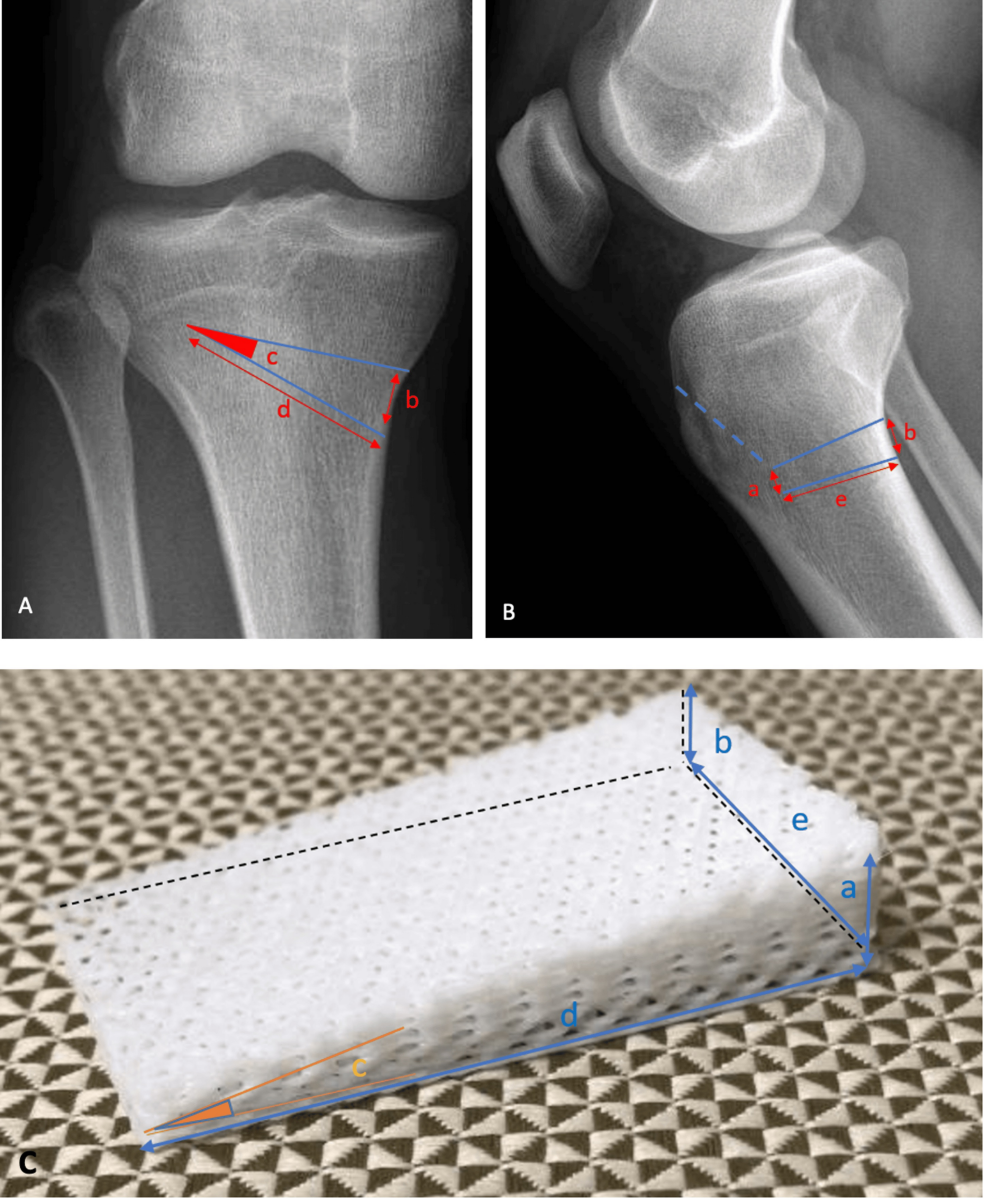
Required dimensions for the bone spacer wedge. A: anteroposterior knee radiograph with the bone wedge dimensions overlayed; B: lateral knee radiograph with the bone wedge dimensions overlayed; C: image of the Osteopore bone wedge used in high tibial osteotomies with labelled dimensions; a: anterior wedge height (conventionally measured as “b-2 mm”; however in anterior cruciate ligament-deficient patients, increased correction may be beneficial), b: posterior wedge height (measured from the medial cortex as the gap required to create the desired mechanical axis correction. We would recommend that 1.2 mm cut be factored in for the thickness of the precision saw blade); c: wedge angle (measured as the lateral most angle of the tibia seen on the anteroposterior radiograph); d: wedge length (measured as the medial to lateral length of the inferior most edge on the anteroposterior radiograph); e: wedge width (measured as 33% to 100% from the anterior cortex (0%) and the posterior cortex (100%) at the pre-designated site of the inferior most edge of the osteotomy on the anteroposterior radiograph).
